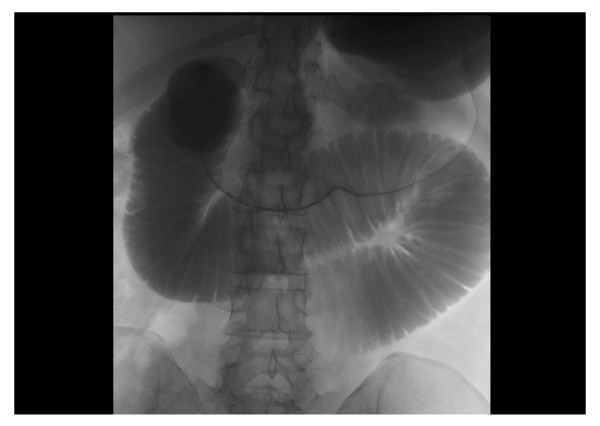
Contrast swallow study, plain radiograph of abdomen 30 minutes after contrast ingestion. Gastrografin contrast study demonstrating proximal jejunal dilatation with an abrupt transition point at the jejunoileal anastomosis. No further contrast passage distally, with associated collapsed small bowel.
